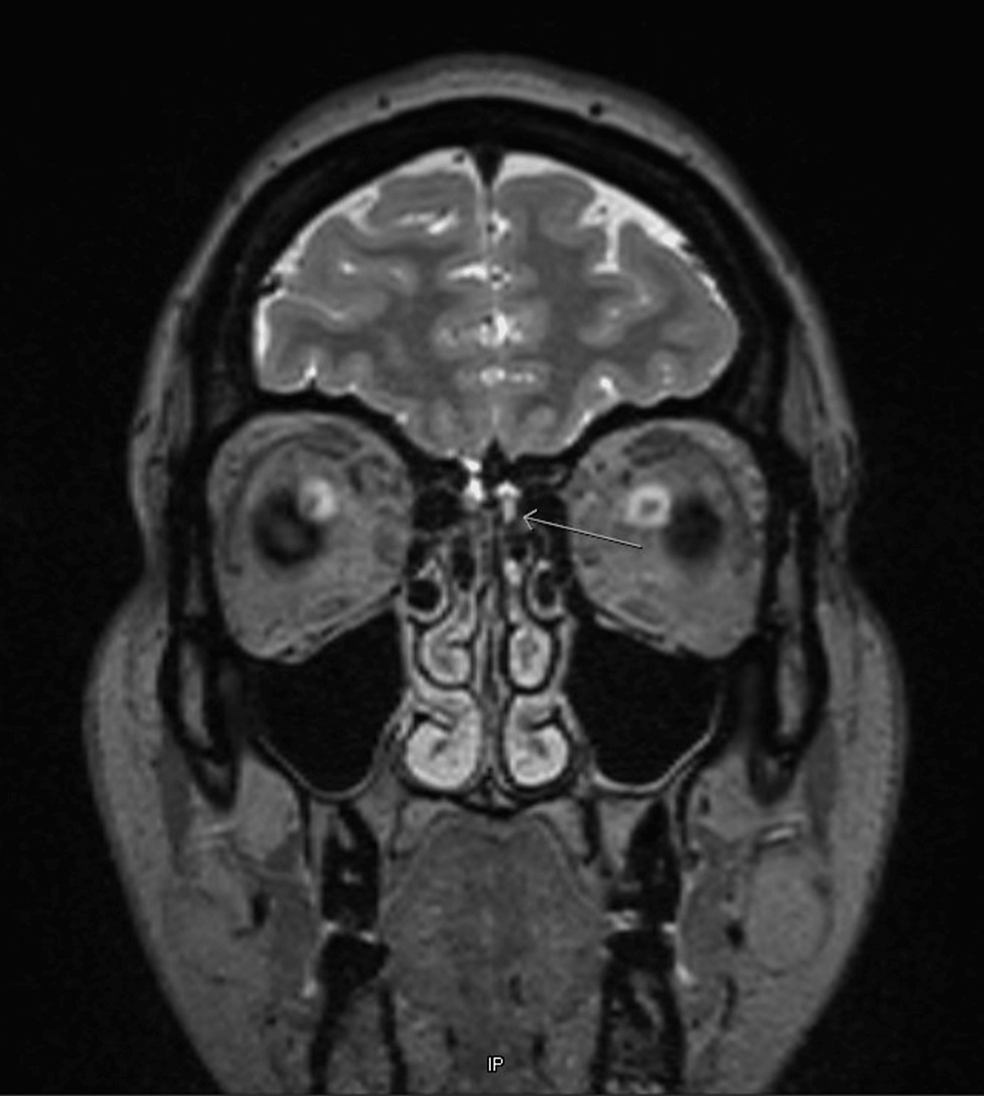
The head CT scan shows a widened empty sella and slightly tortuous optic nerves with a prominent perioptic CSF space.
